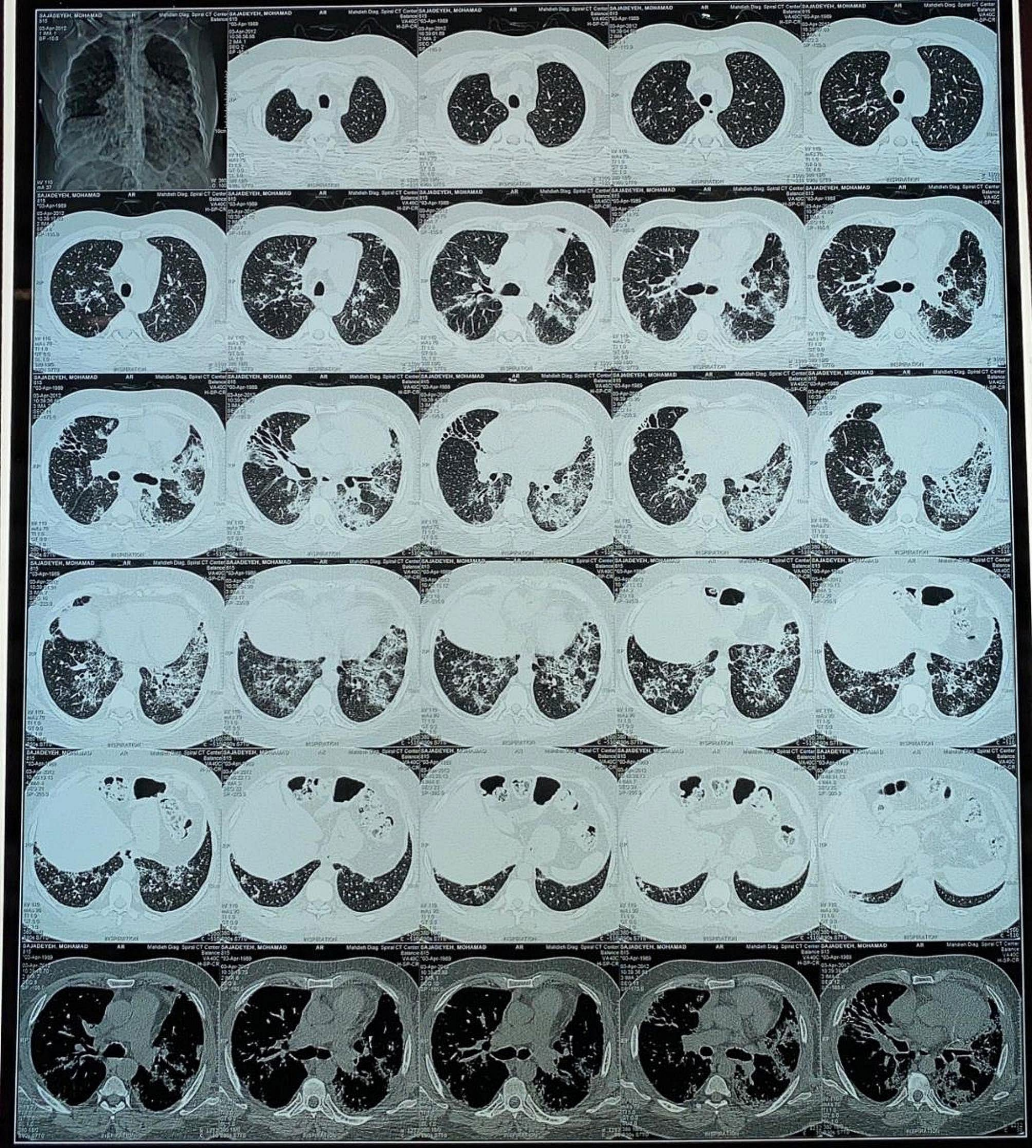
Some CT scan lesions disappeared after discontinuation of sirolimus
